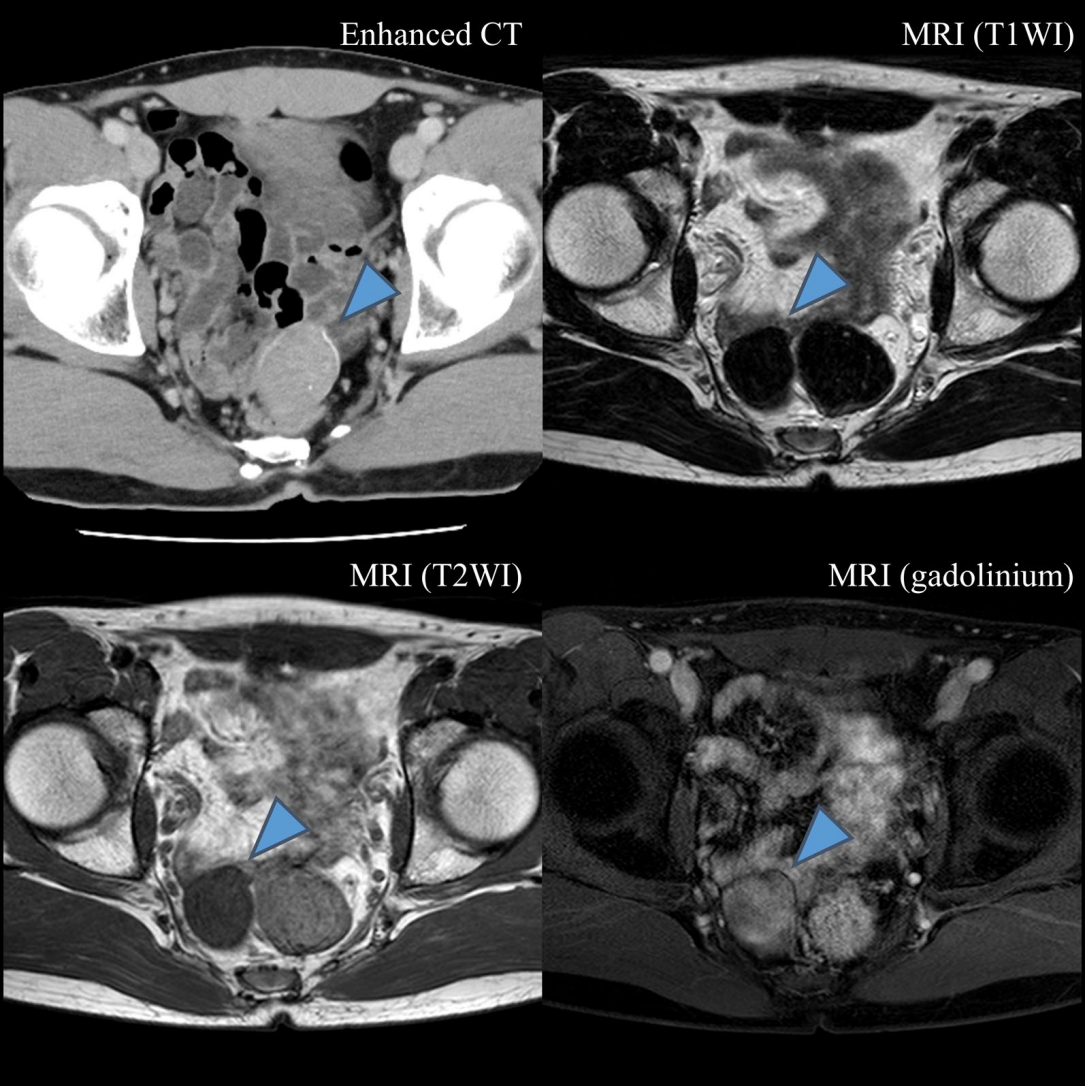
CT and MRI images. A 45-mm-sized mass (blue arrow head) with partial calcification was observed in the ileum on enhanced CT. The mass was hypointense signal on both T1WI and T2WI, and isointense signal on gadolinium-enhanced T1WI. *CT* computed tomography, *MRI* magnetic response image, *T1WI* T1-weighted image, *T2WI* T2-weighted image
